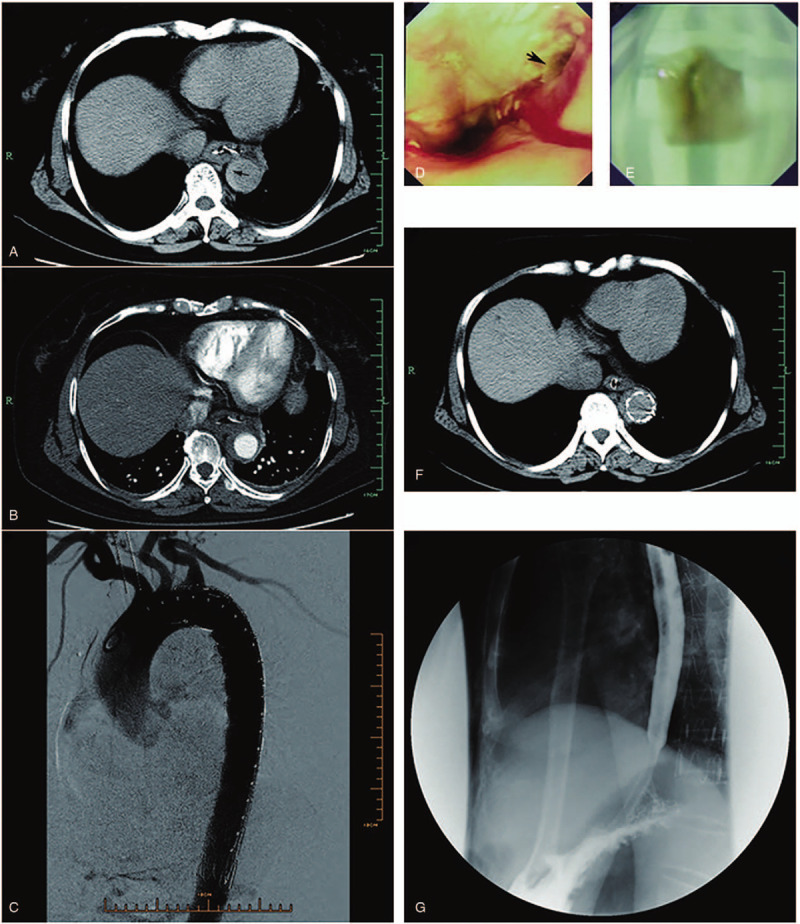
Representative images of case 3. (a) Chest CT was performed and revealed a lower esophageal foreign body and crescent wall thickening in the descending aorta (arrow). (b) Subsequent CTA demonstrated a descending IMH. (c) An endograft (34 × 200 mm, Lifetech Scientific, China) was successfully placed inside the descending aorta. Postoperative angiography confirmed successful results. (d) Endoscopy showed a foreign body (arrow) in the lower third of the esophagus, stabbing into the esophageal wall. Esophageal mucosal injury and fresh bleeding was also observed. (e) The removed duck bone (2 × 3 cm). (f) The chest CT (August 26, 2015) revealed no sign of mediastinal infection. (g) Esophagography (August 27, 2015) with diatrizoate meglumine test was negative.
